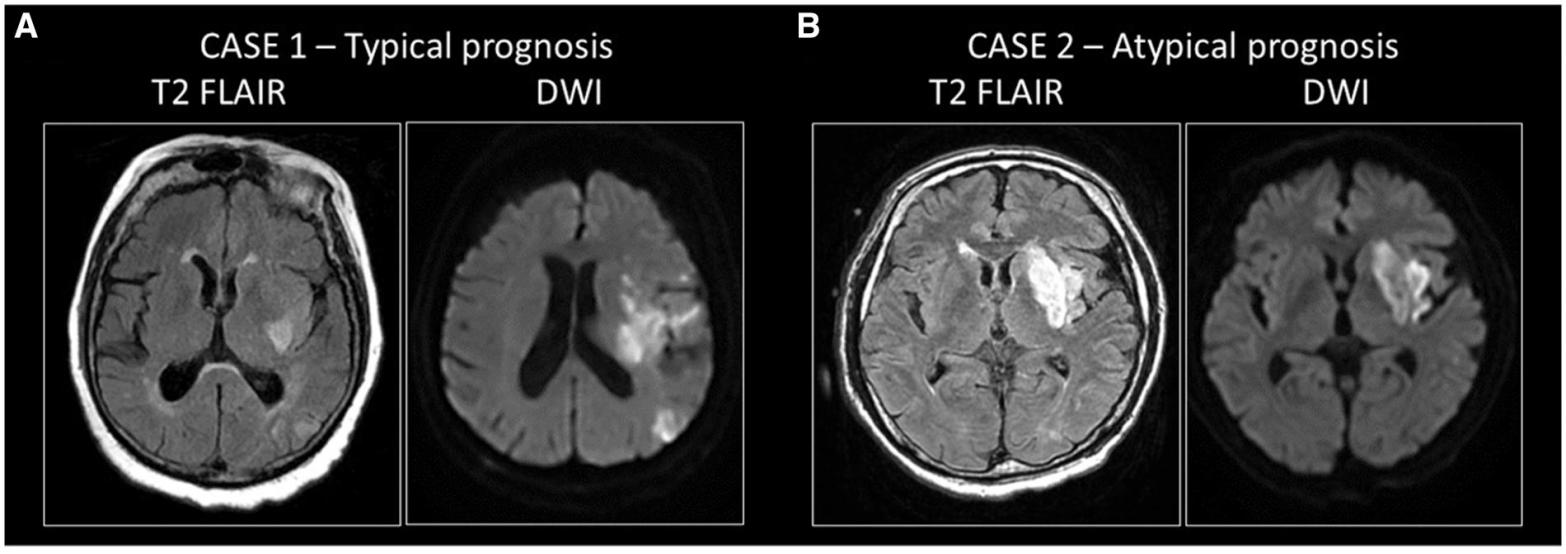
Clinical cases of typical and atypical prognosis in patients with ischemic stroke. (A) Case 1: typical prognosis in a patient with a large infarct volume with severe residual symptoms. An 81-year-old female patient was admitted with aphasia and severe right-sided hemiparesis. The National Institutes of Health Stroke Scale score at the initial examination was 14 points. The Alberta Stroke Programme Early CT score was 9/10. CT angiogram showed occlusion of the left middle cerebral artery (M1). The onset of symptoms was unknown. According to protocol, CT perfusion was performed, showing a large salvageable penumbra without core. Despite the favorable initial imaging results, after successful clot removal (eTICI 3), control MRI showed the development of a large infarct on both diffusion-weighted imaging (DWI) and FLAIR. National Institutes of Health Stroke Scale score at discharge was 22 points. The 90-day modified Rankin Scale (mRS) score, which is based on disability and dependence on activities of daily living following the stroke, was 5; the patient had severe residual symptoms. (B) Case 2: atypical prognosis in a patient with moderate infarct volume and severe residual symptoms. A 65-year-old male patient presented with aphasia and right-sided hemiparesis. The NIHSS at admission was 9 points. Alberta Stroke Programme Early CT Score score was 8/10. A CT angiogram showed occlusion of the left middle cerebral artery (M2). The onset of symptoms was unknown. According to protocol, CT perfusion was performed, showing a 54 ml penumbra and a small 4 ml core. Endovascular treatment was indicated and successfully performed (eTICI 3). Severe clinical progression was noted, with NIHSS 16 points at discharge, and a 90-day mRS of 5 points. The clinical data presented here were not collected specifically for this project. These patient data were selected for representative purposes for this article and were obtained from the database of the Department of Neurology, Albert Szent-Györgyi Health Centre, University of Szeged, under the supervision of Dr Nikoletta Szabó.
